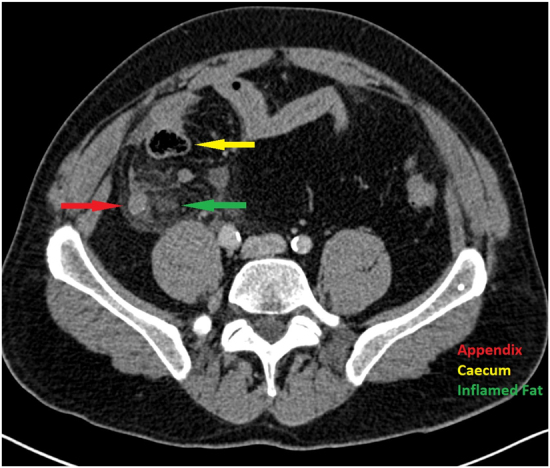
CT scan showing appendix and inflamed fat. Finding the appendix during surgery can be a challenge when inflammation obscures anatomical planes.
